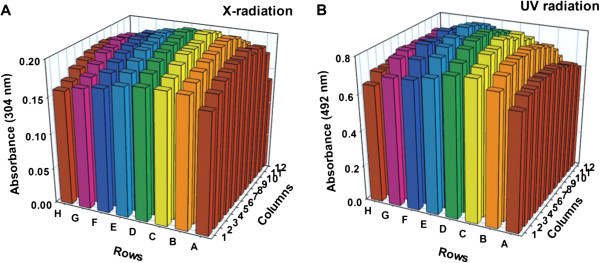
X- and UV-radiation dose rates within the 96 wells of a microtiter plate. (A) The absorbance within each well was determined at 304 nm (A_304_) in triplicate experiments using a chemical dosimeter and plotted. The rows (letters) and column (numbers) in the graph associate data with individual wells in the microtiter plate. The average dose rate for 160 kV X-rays was determined from (ΔA_304_)(280 Gy min^-1^) to be 17.96 Gy/min (sd = 0.02), and plates received a dose of 54 Gy. (B) A_492_ values for resazurin absorbance (indicating the cellular metabolic activity within each well) were determined from 6 experiments using a bioassay. Plates received a UV radiation dose of 50 J m^-2^. Bioassay A_492_ values were averaged and plotted. The mean value over 96 wells was 0.77 (sd = 0.02) A_492_ units.
